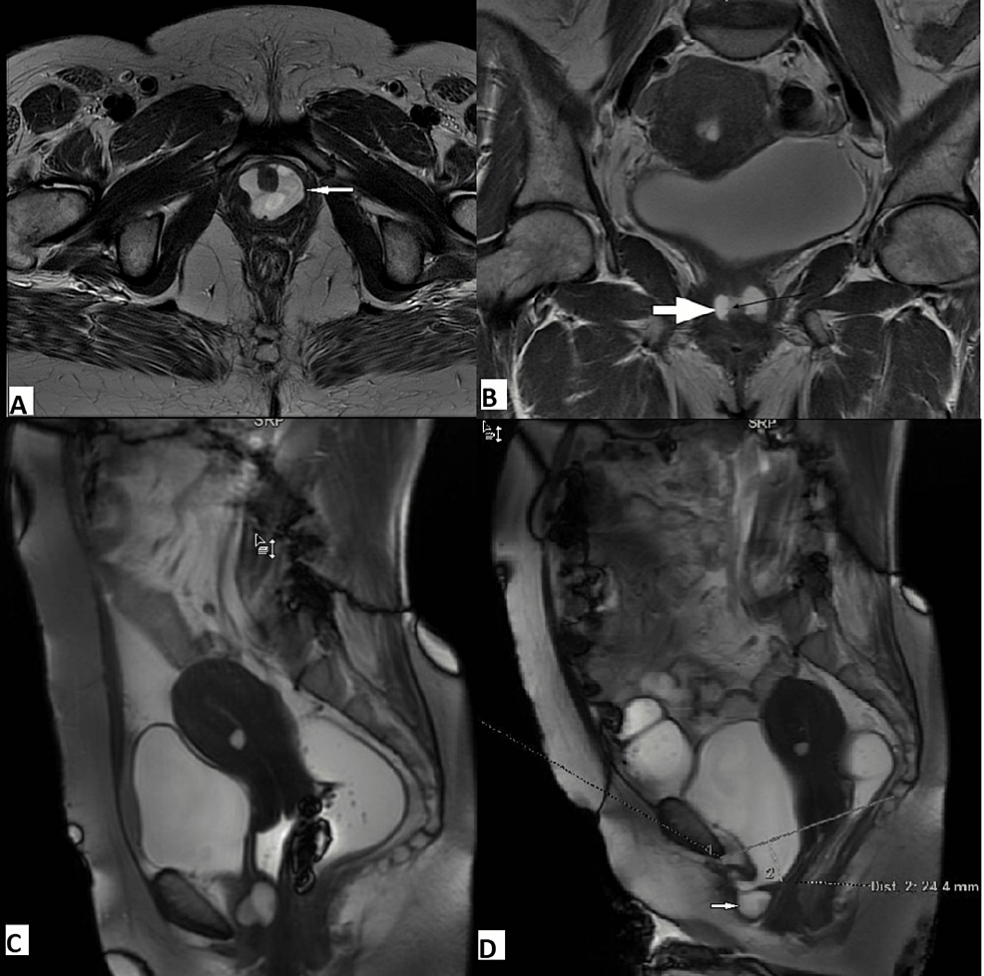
A 46-year-old lady with urethral diverticulum and cystocele. T2 axial (A) and coronal (B) images reveal saddle-shaped fluid collection (white arrow) around the urethra (black arrow), indicating urethral diverticulum. Midline sagittal dynamic FIESTA MRI in rest phase (C) reveals urethral diverticulum with the normal position of the bladder base and urethra. Image in strain phase (D) reveals cystocele with urethral hypermobility. The urethral diverticulum is seen anteriorly (white arrow). FIESTA: fast imaging employing steady-state acquisition.
